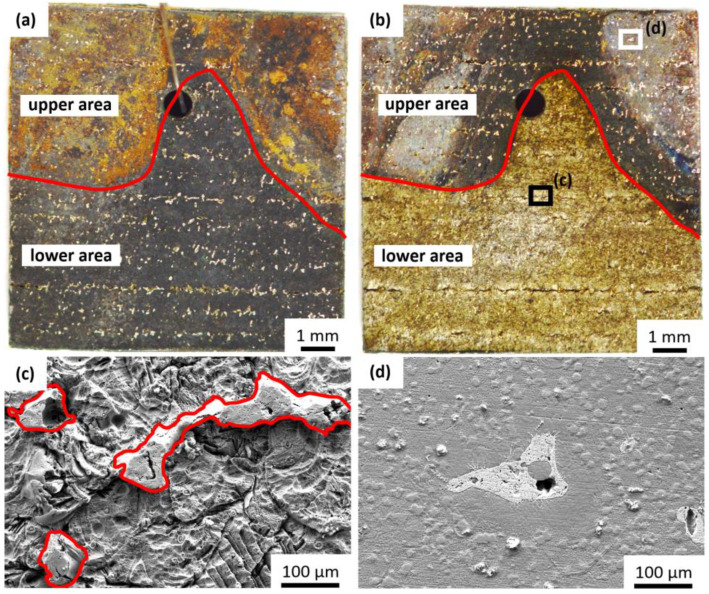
Local differences in degradation progress, FeMnAgCaLa after 1344 h (37 °C, RL, aeration): (a) image before ultrasonic cleaning; (b) image after ultrasonic cleaning; (c) SEM-SE: Magnified section of black box in (b); (d) SEM-SE: Magnified section of white box in (b).
